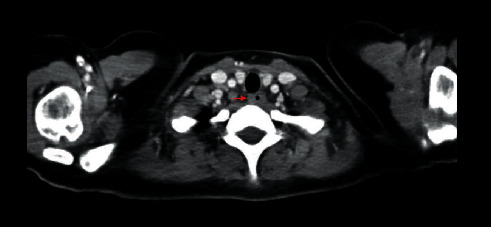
CT chest/abdomen/pelvis demonstrating diffuse, circumferential esophageal thickening as denoted by red arrow.
